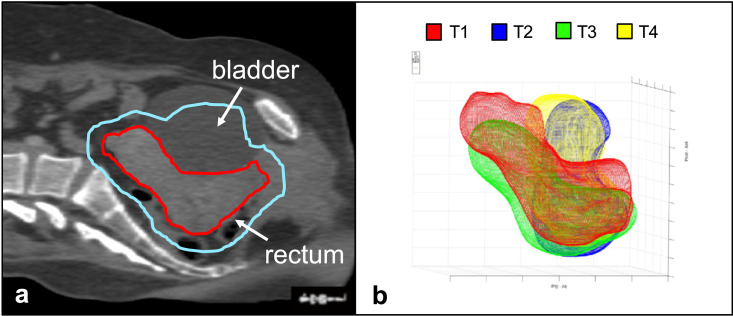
(a) Pre-treatment planning computed tomography image of a cervical cancer patient with the clinical target volume outlined in *red* and the planning target volume outlined in *cyan*. Note that large portions of healthy tissues such as the bladder and rectum are included in the planning target volume. (b) Superimposition of 3-D uterine contours of the cervical cancer patient in (a) derived from ultrasound images taken at four different time points (T1–T4) over the course of radiotherapy treatment. Note the large amount of day-to-day motion and deformation of the uterus over the course of radiotherapy treatment.
